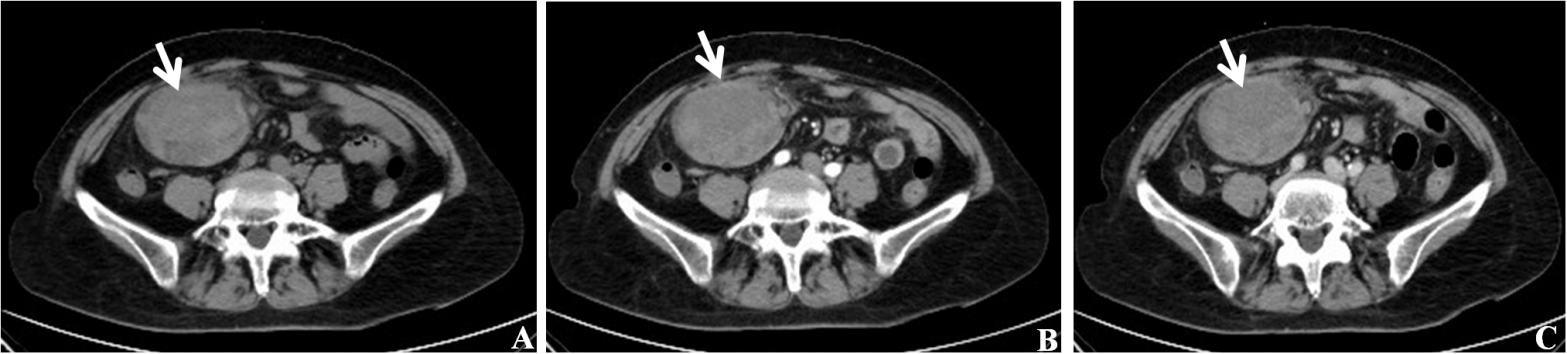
Radiographic characteristics of the extraovarian adult granulosa cell tumor of the greater omentum. (A) Abdominal CT scan demonstrated a soft tissue mass within the right abdominal cavity(indicated by the white arrow). The mass exhibited regular and indistinct border and heterogeneous density, containing irregular patchy areas with mild hyperdensity. (B, C) Post-contrast images demonstrate heterogeneous enhancement of the lesion(indicated by the white arrow), while the previously noted hyperdense areas exhibit no significant enhancement. These findings were suggestive of a neoplastic lesion with associated hemorrhage. (B) Arterial Phase, (C) Venous Phase.
